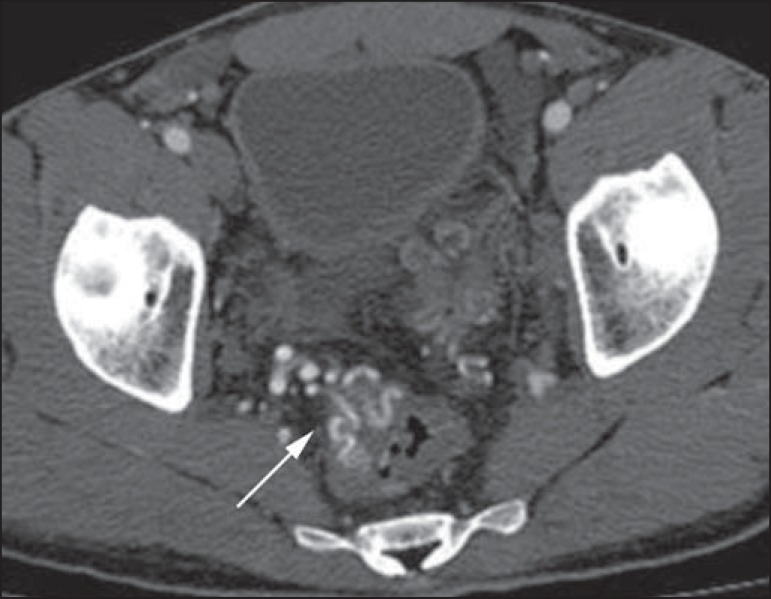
CT angiography, arterial phase in cirrhotic patient, with portal
